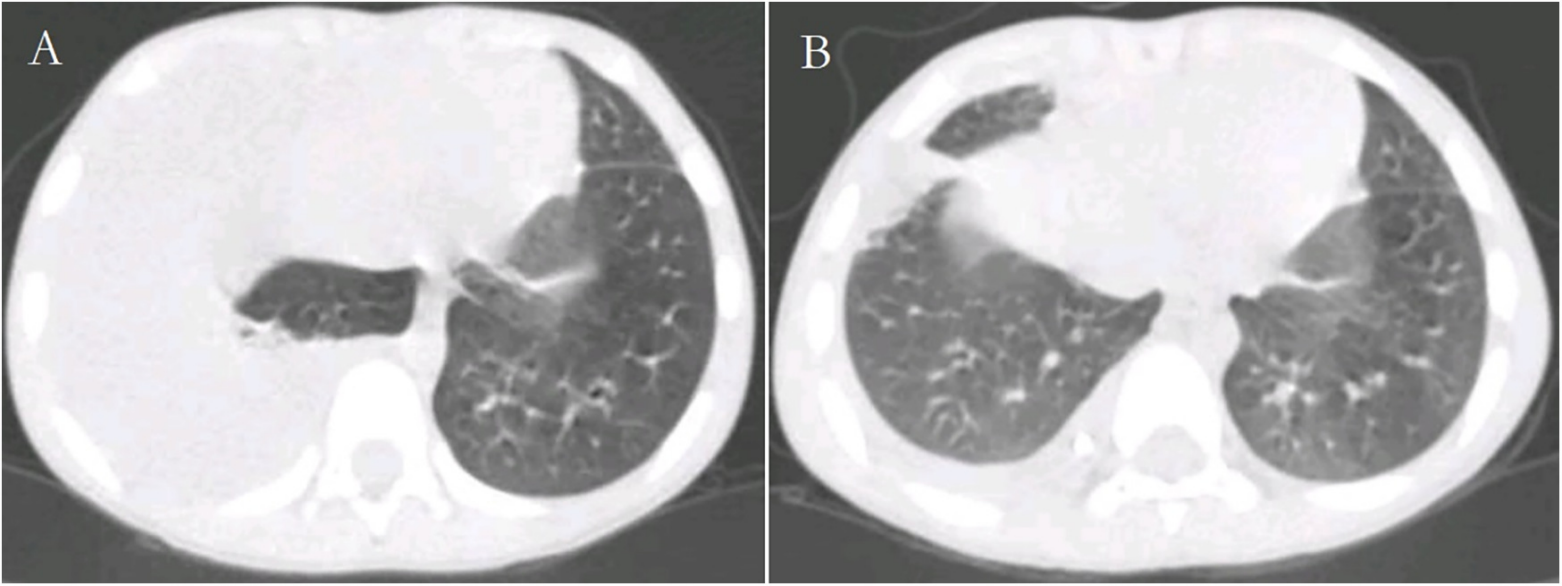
Chest CT (lung windows) of the patient before (A) and after (B) treatment.
